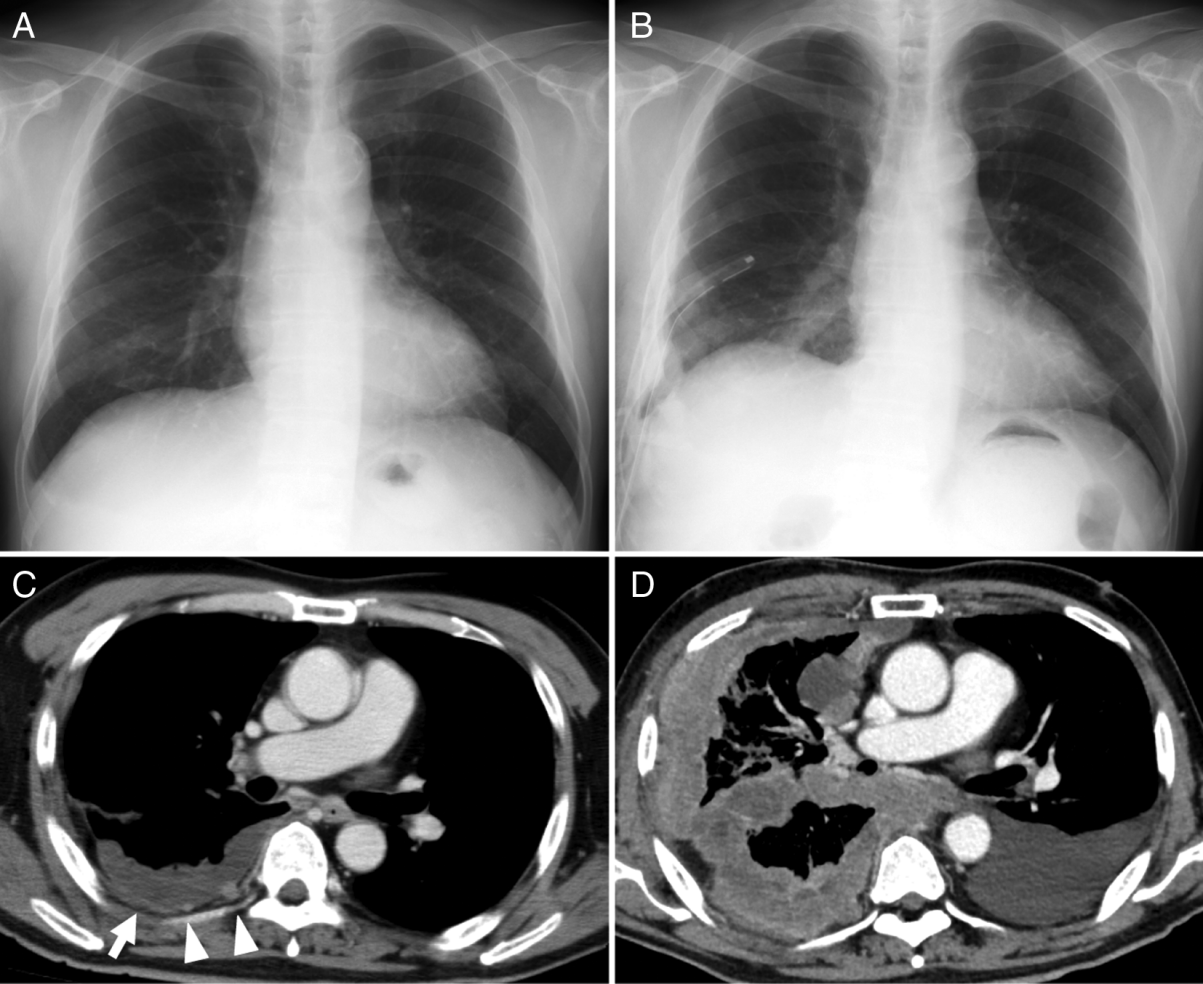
(A) Normal chest X‐ray performed 4 months prior to referral. (B) Chest X‐ray performed 1 month prior to referral, after the insertion of thoracic drainage, showing an infiltration of the right lower lung field with blunting of the costophrenic angle. (C) Thoracic CT performed 1 month prior to referral. Arrowhead depicts the presence of small amounts of right pleural effusion with enhancing parietal pleural thickening or tiny nodules. (D) Thoracic CT on referral depicts the emergence of a large inhomogeneously enhancing pleural thickening measuring up to 3.4 cm and extending to the entire right hemithorax, together with left‐sided pleural effusion. CT, computed tomography.
